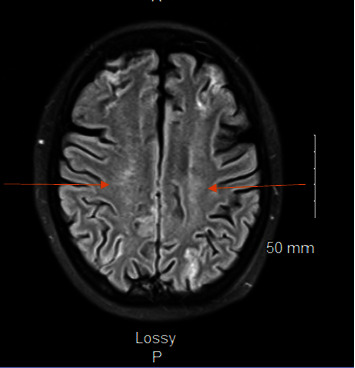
MRI of patient's brain showing global ischemia. This brain image shows an image cut from the patient's MRI that was ordered as with and without contrast of the brain. Global ischemic changes are noted by the orange arrows noted at the watershed areas.
